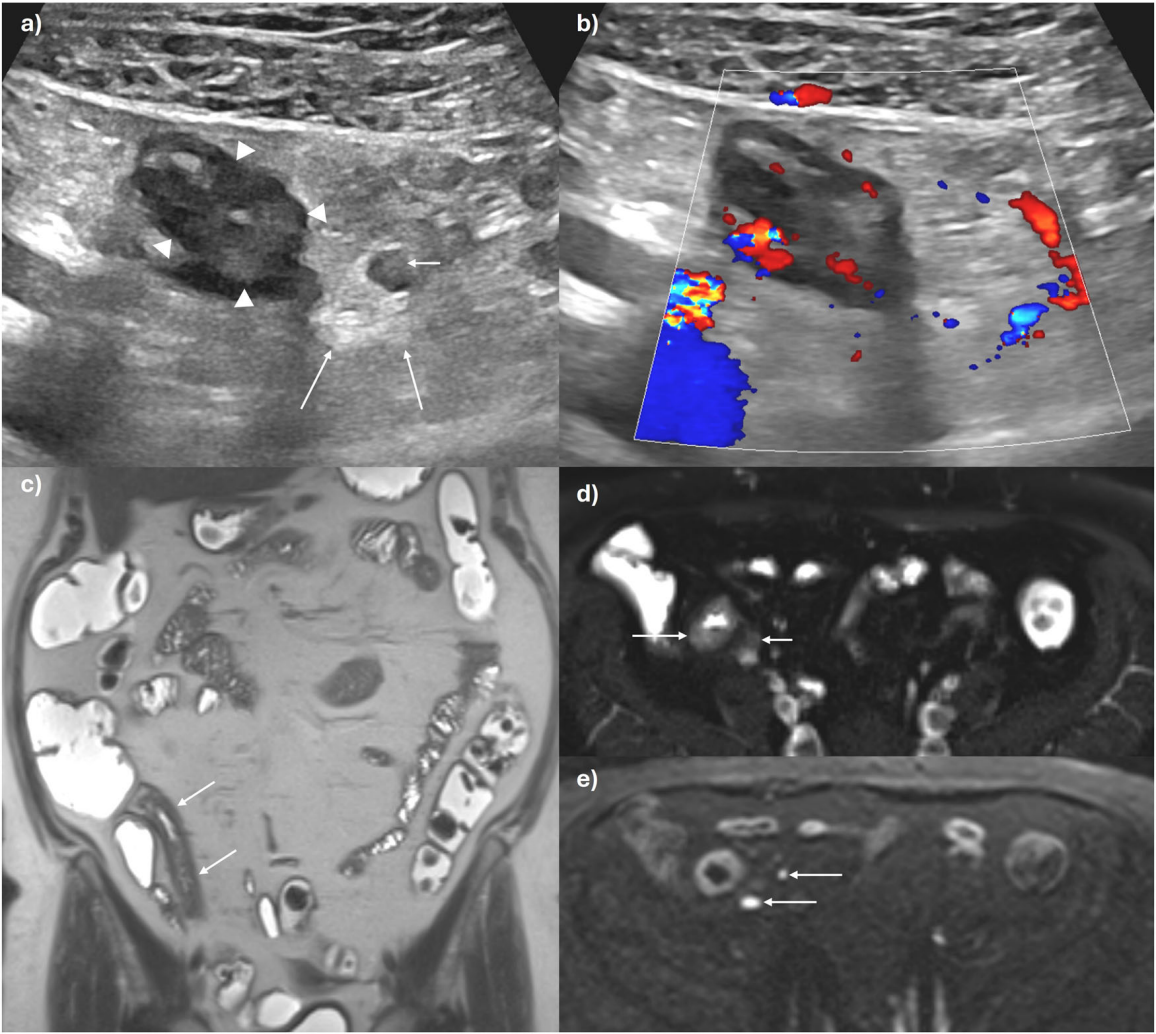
A 59-year-old female with a new diagnosis of CD. a IUS shows a thickened terminal ileum with extensive loss of mural stratification (arrowheads), hyperechoic mesenteric fat (long arrows), and an enlarged mesenteric lymph node (short arrow). b There is increased vascularity in the bowel wall on colour Doppler extending into the inflammatory fat. c Coronal T2-weighted image again shows the thickened terminal ileum (arrows). d Mural oedema (long arrow) and perimural inflammation (short arrow) in the terminal ileum are clearly depicted on this axial T2-weighted image with fat saturation. e Axial diffusion-weighted image (b600) demonstrates restriction in the inflamed terminal ileum with adjacent lymph nodes (arrows)
